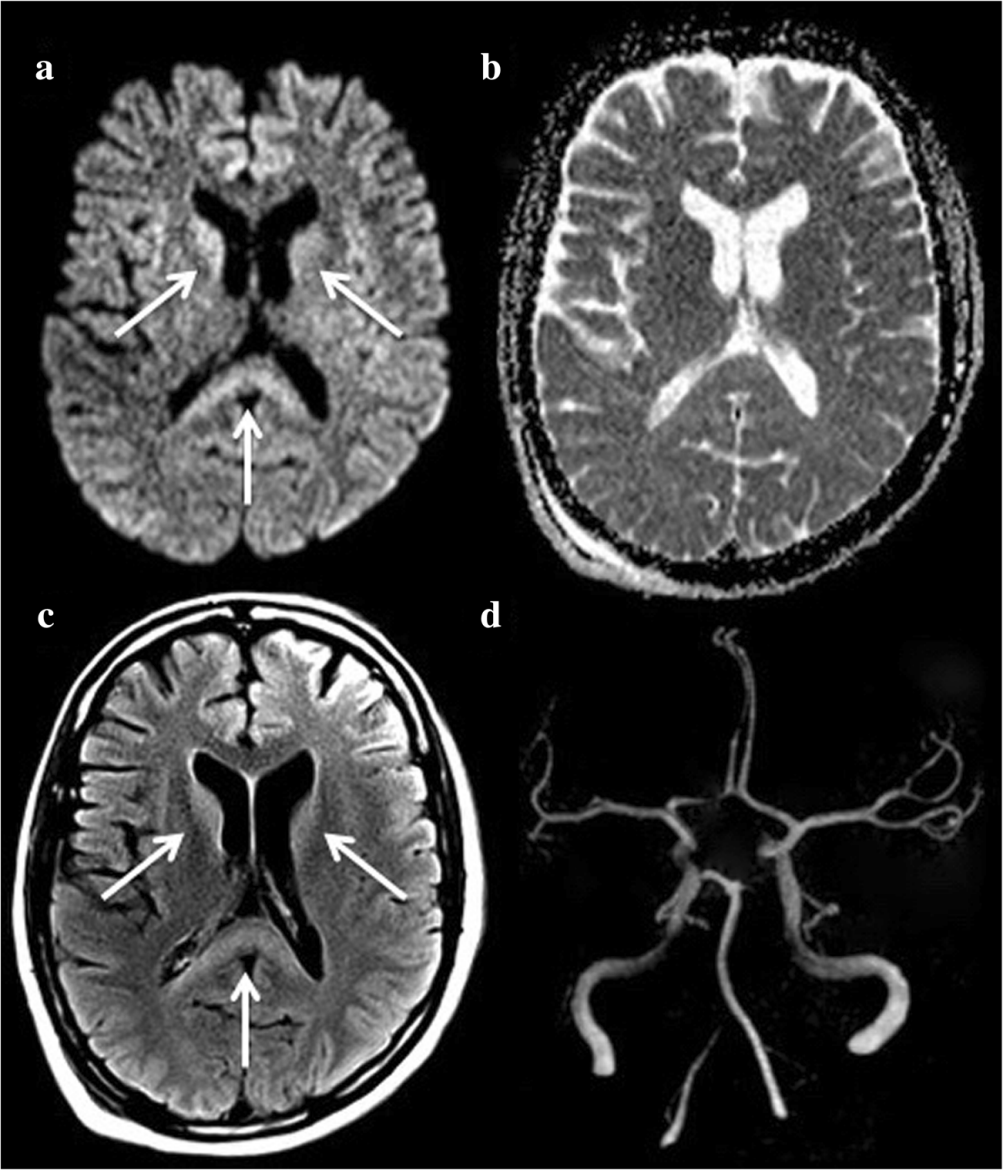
A 42-year-old woman with hypoglycaemia presenting with disturbance of consciousness after abdominal surgery. DWI shows hyperintensities in the basal ganglia and the splenium of the corpus callosum (a, c, arrows) with no restriction (b) and no arterial occlusion (d)
